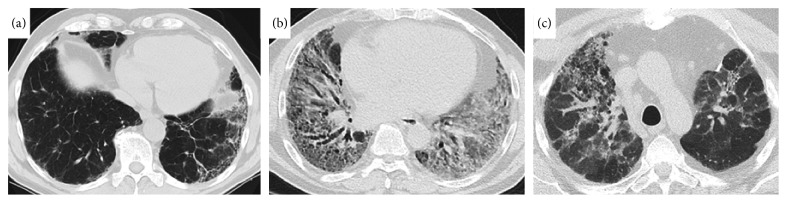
(a) Radiographic probable UIP pattern (Patient 1). (b) Radiographic NSIP pattern (Patient 11). (c) Radiographic HP pattern (Patient 10). HP: hypersensitivity pneumonitis, NSIP: nonspecific interstitial pneumonia, and UIP: usual interstitial pneumonia.
